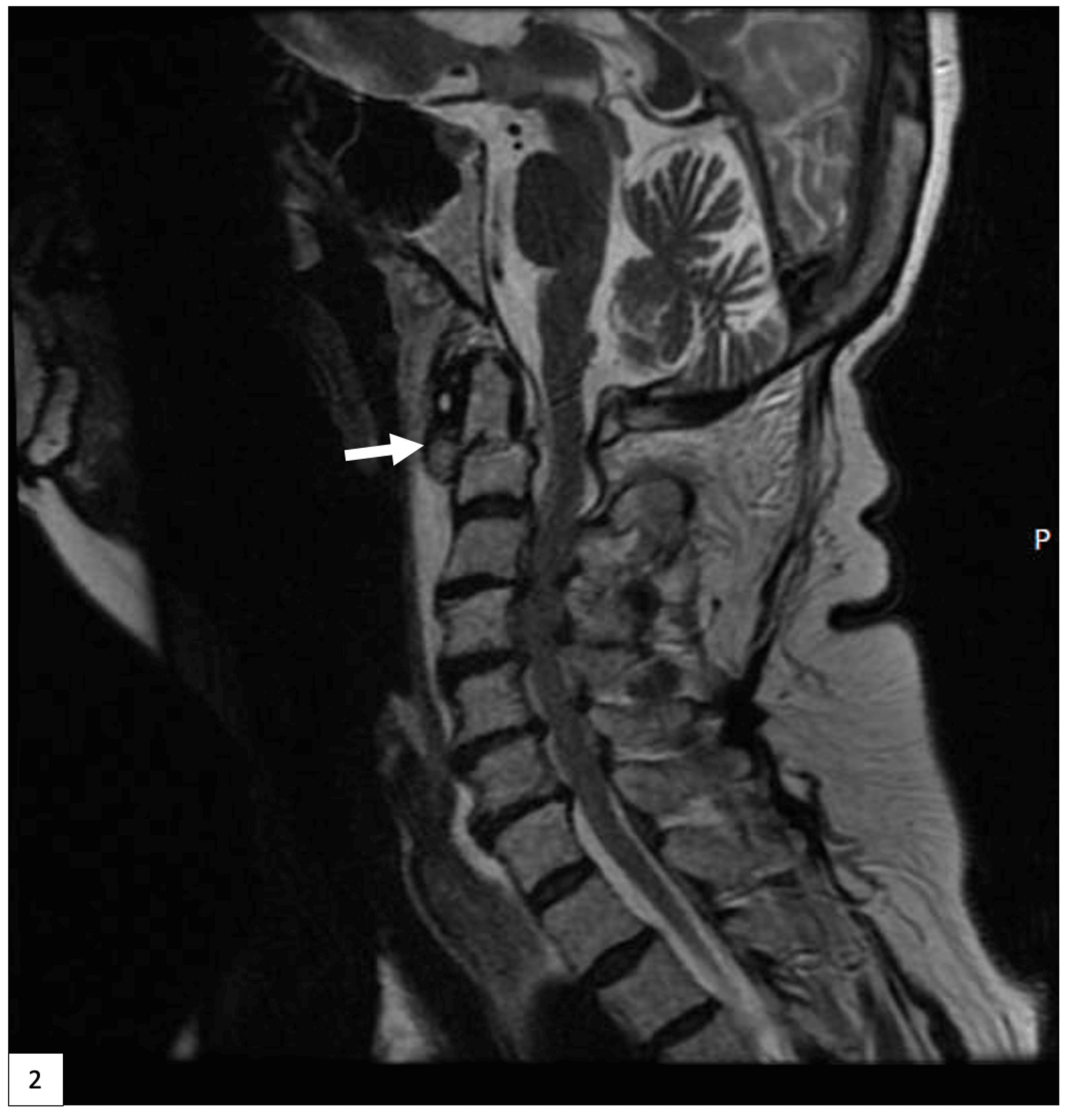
T2 sagittal MRI of the cervical spine demonstrating the type III dens fracture indicated by the white arrow MRI: Magnetic resonance imaging
